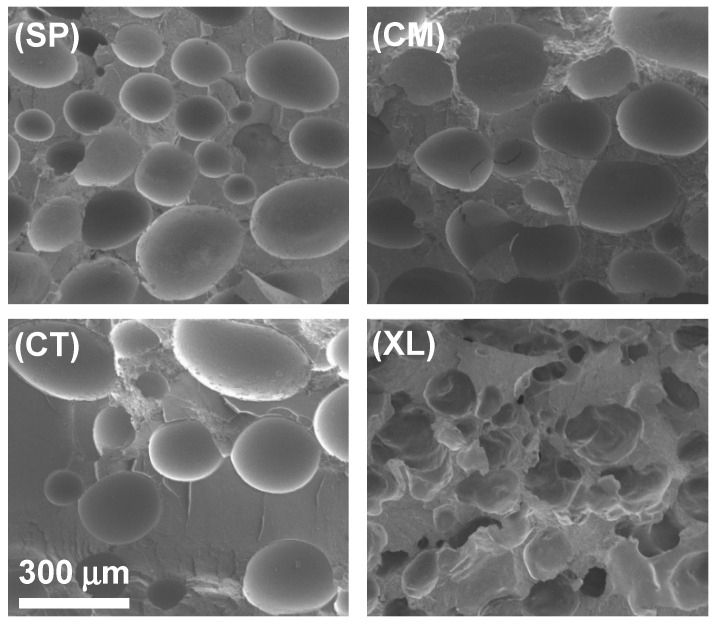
SEM images of SSSP-processed (SP), cryomilled (CM), manually blended control (CT), and crosslinked/cryomilled (XL) PLA foams with 6.5 wt% CFA.
